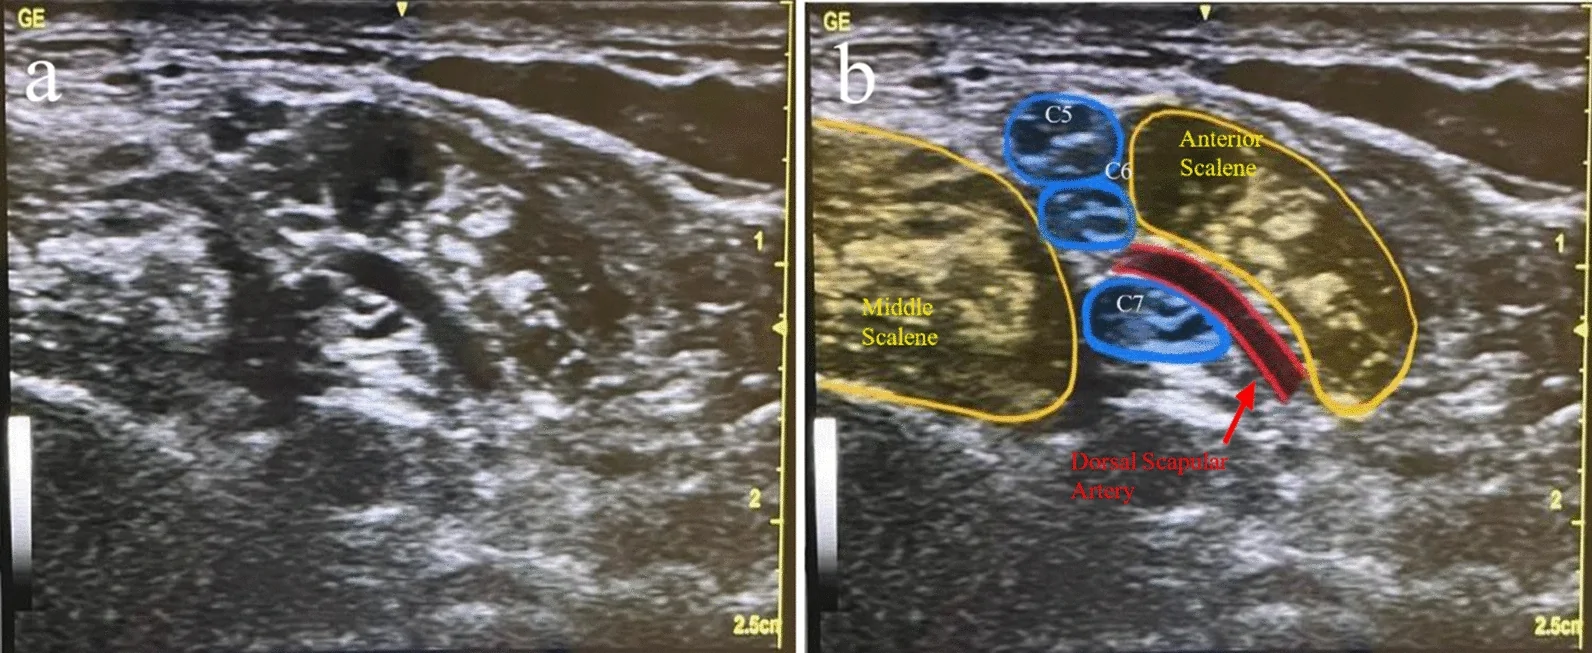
Unannotated (a) and annotated (b) ultrasound representation of DSA traversing between C6 and C7 nerve roots and impinging on the C7 nerve root
